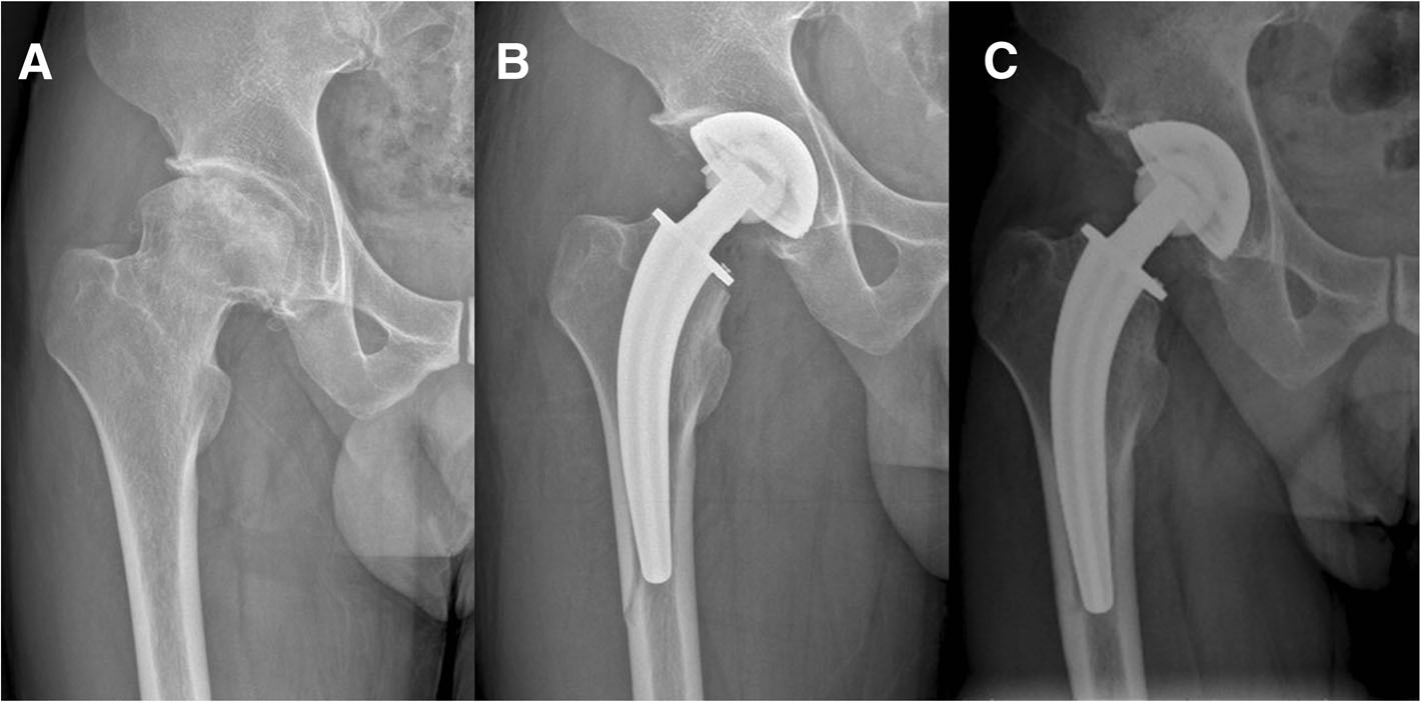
Preoperative anteroposterior radiograph of a 30-year-old man showing femoral head osteonecrosis of the right hip (a). Anteroposterior radiograph after THA using CFP stem showing PPF of the lateral femoral diaphysis (b). Anteroposterior radiograph at 1-year follow-up showing healing fracture (c)
